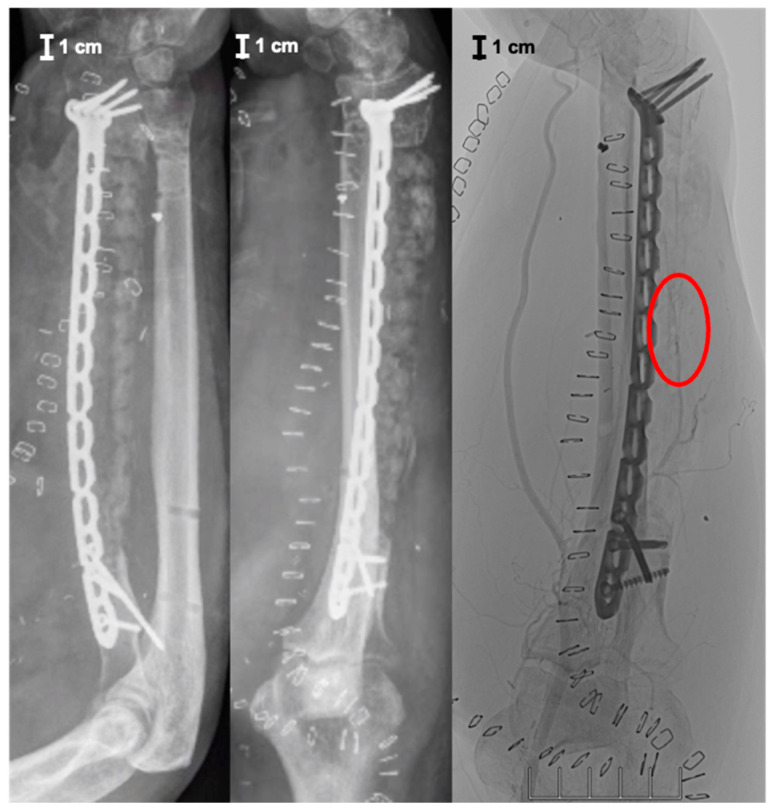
Postoperative X-ray images (AP and lateral view) and digital subtraction angiography (DSA) of the right forearm showing adequate implant position and graft vascularization (red frame).
